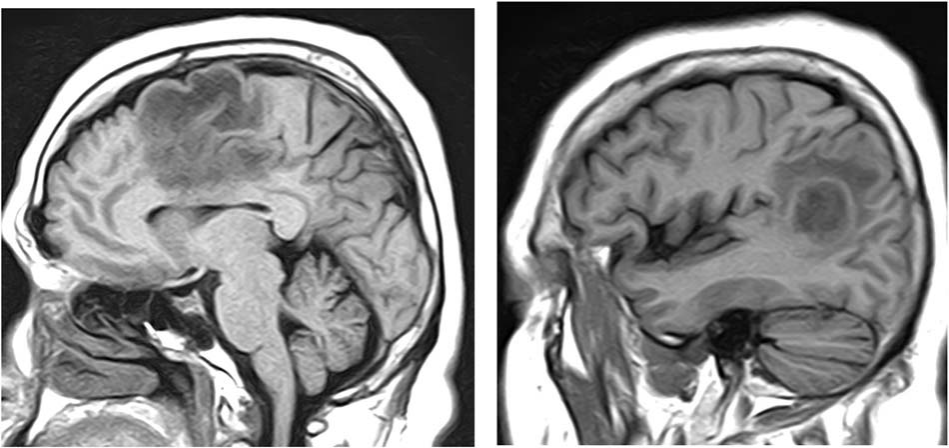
MRI of brain without contrast showed two enhancing masses in the left frontal and left parieto-occipital lobes, 2.1 cm × 2.7 cm × 1.9 cm and 1.9 cm × 2.0 cm × 2.5 cm, respectively.
